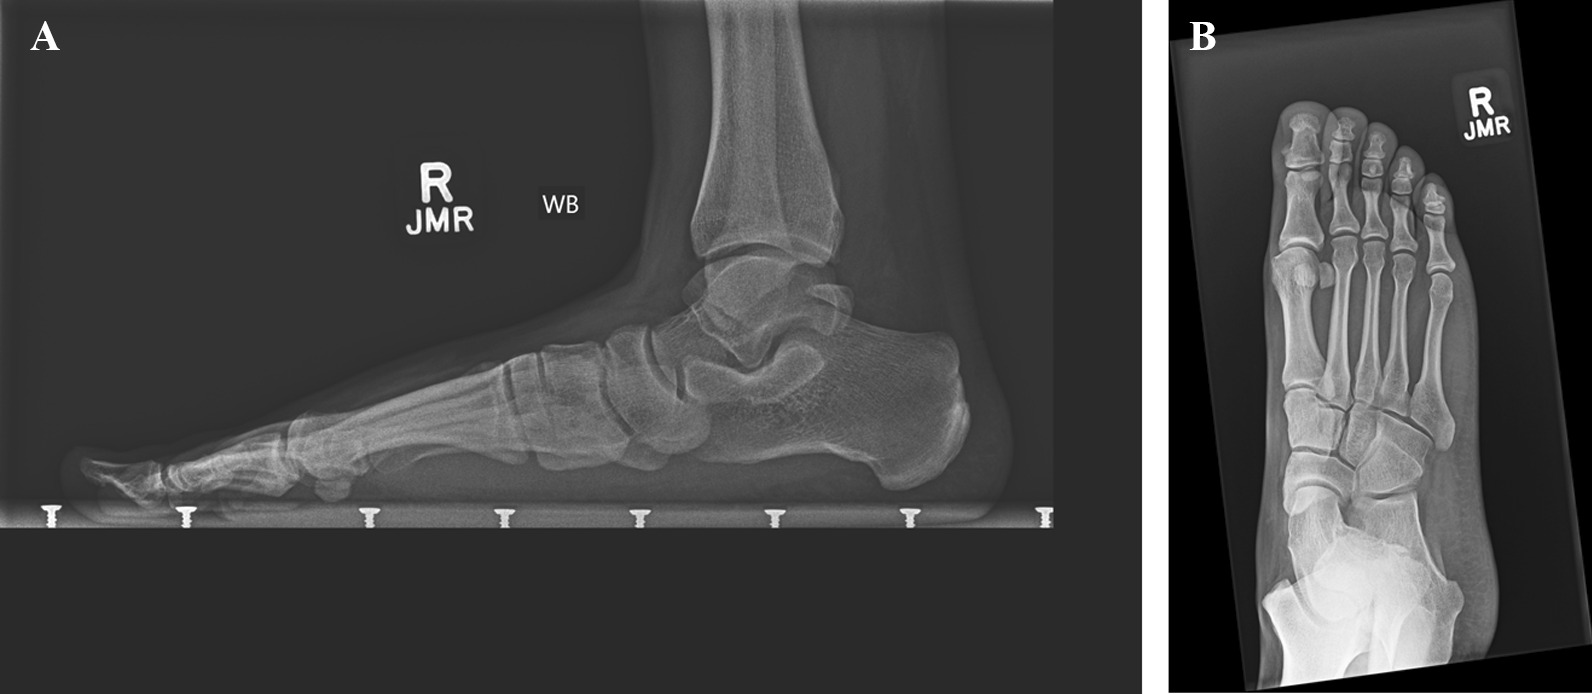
A Sagittal view CT of a medial TC coalition. B Coronal view CT of bilateral TC coalitions, seen only on the left in this view
